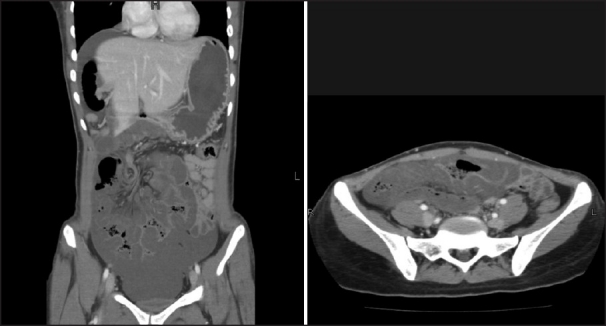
CT scan images showing dilated small bowel loops, significant free fluid and intramural air
